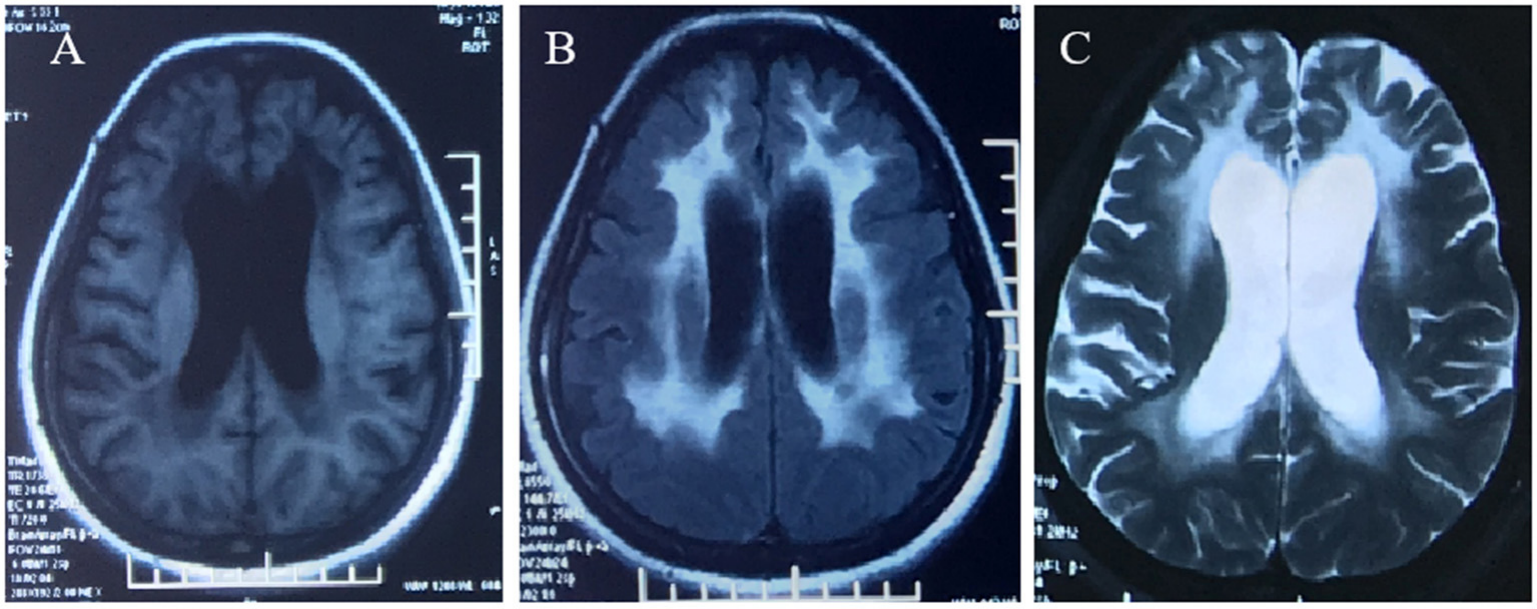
Brain MRI demonstrated diffuse, symmetrical abnormal signal in the bilateral cerebral white matter, which was low signal in T1WI (A), high signal in FLAIR (B), and high signal in T2WI (C).
